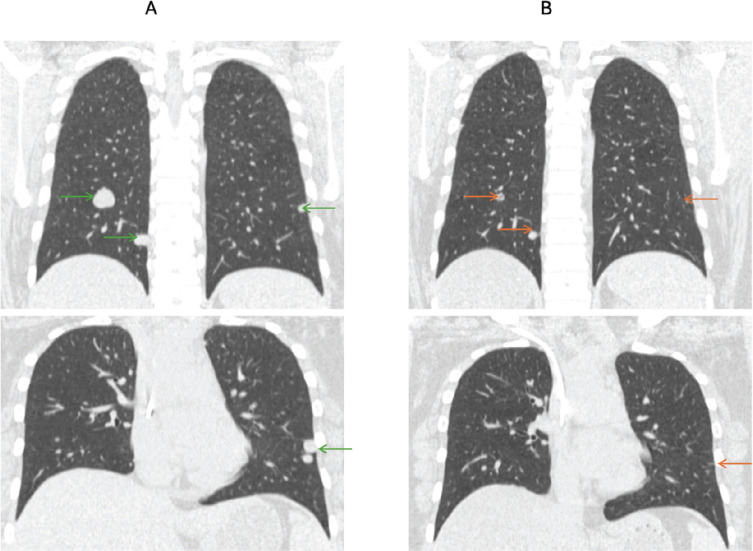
(a): Pre-treatment computed tomography images of a patient with metastatic ASPS with lung metastasis (green arrows). (b): Computed tomography images after 12 months of atezolizumab show significant reduction in previously seen lung nodules (red arrows).
